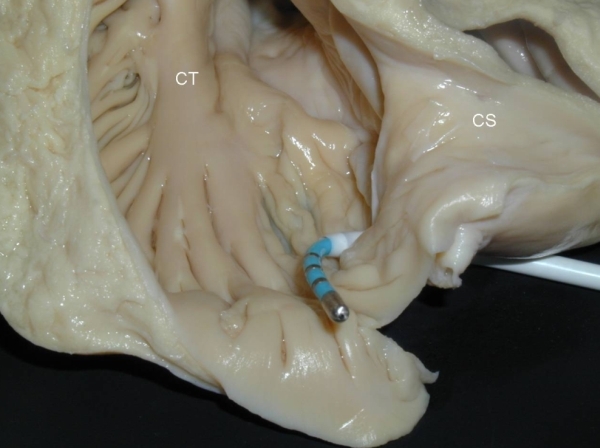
Dissection of an autopsied heart showing left anterior oblique-like view looking through the tricuspid valve into the right atrium with an ablation catheter and guiding sheath placed across the CVTI.  Note the large pectinate muscles emanating from the crista terminalis (CT).  Many pectinates are seen encroaching onto the CVTI and traversing the isthmus into the coronary sinus (CS).
